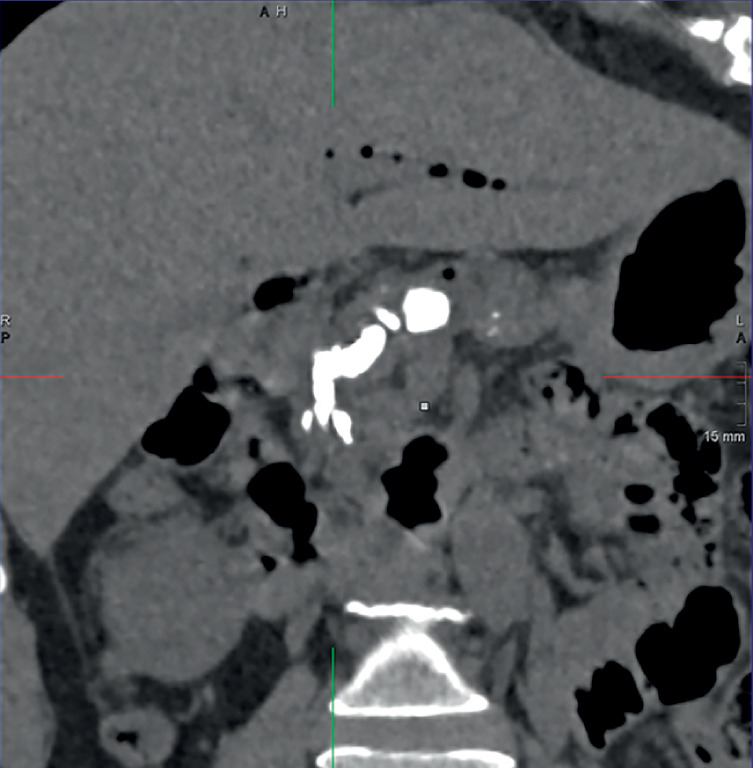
Computed tomography showed chronic pancreatitis with large calcifications, upstream main pancreatic duct dilation, and pneumatosis in the biliary and pancreatic ducts.
